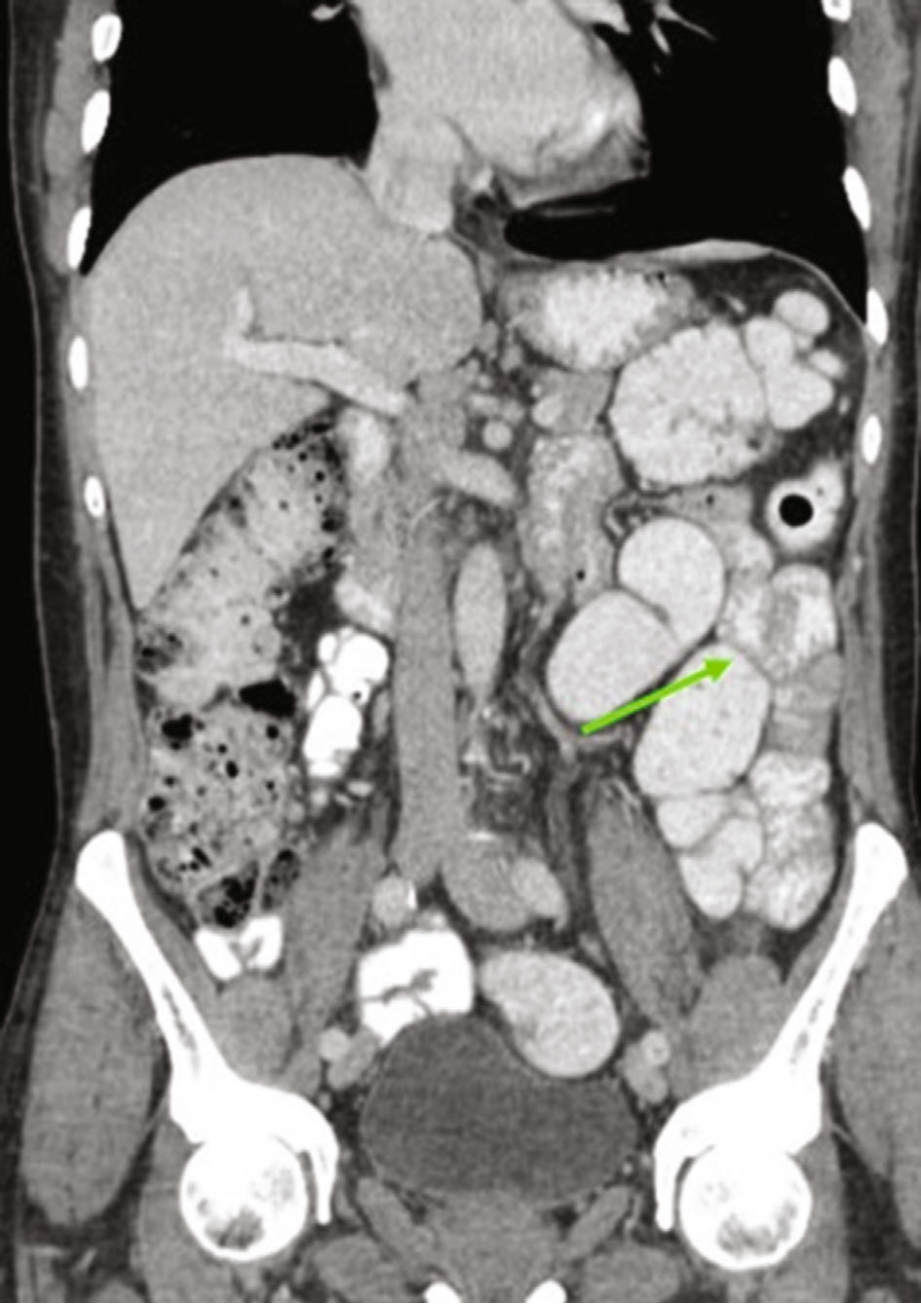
CT abdomen and pelvis with IV, oral, and rectal contrasts showing the jejunal loops project lateral to the sigmoid in the left lumbar region (green arrows).
